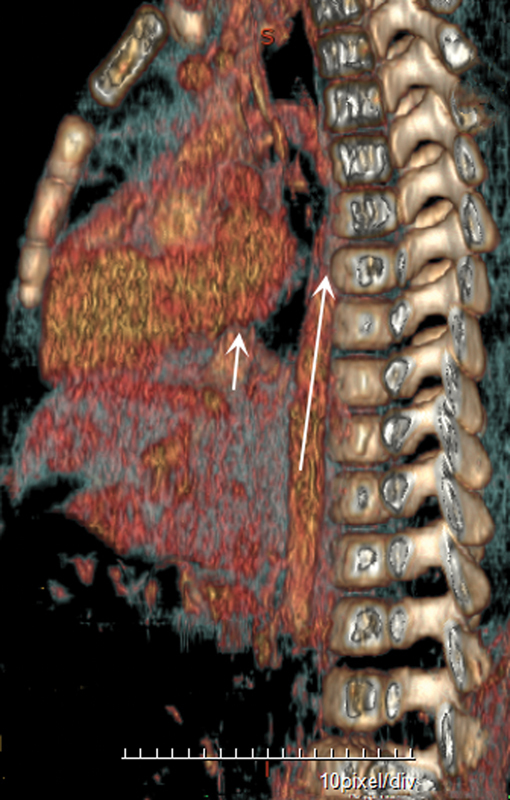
Contrast-enhanced computed tomography on sagittal view showed a hepatic segment of the inferior vena cava draining hepatic veins (
